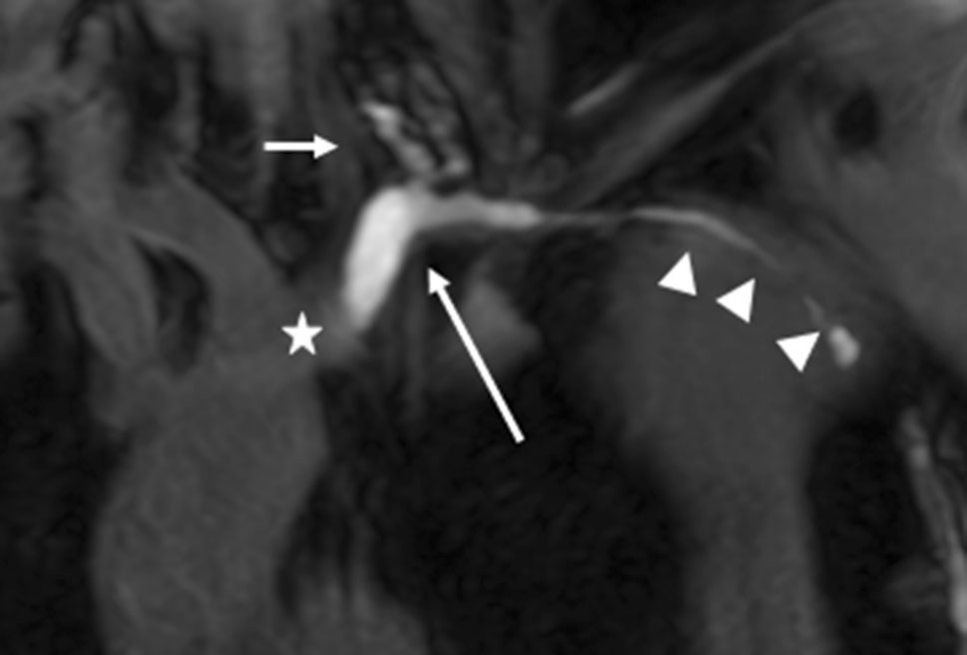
Coronal, T1-weighted MR-lymphangiogram demonstrating enhancement of the terminal thoracic duct (short arrow) draining into the left subclavian vein (long arrow) isolated by proximal and distal thrombotic occlusion (central occlusion: asterisk). From this isolated venous segment the contrast agent drains through the former port catheter tract (arrow heads) to the skin
